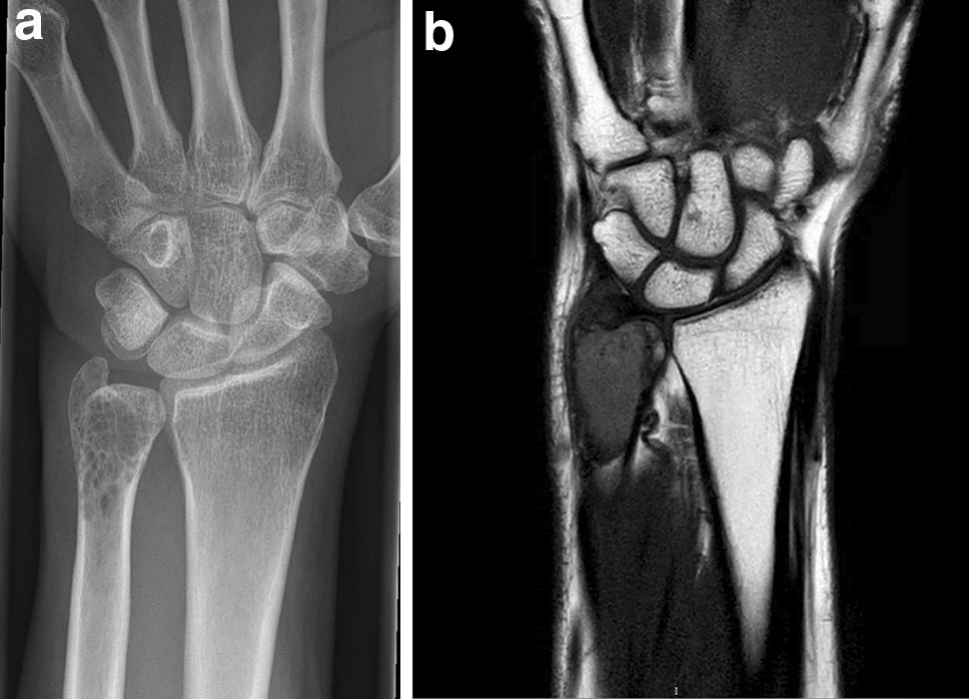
Imaging of a clear cell chondrosarcoma in a VHL patient. a X-ray of the left forearm. The lesion is located in the distal ulna. b Magnetic resonance imaging of the lesion
